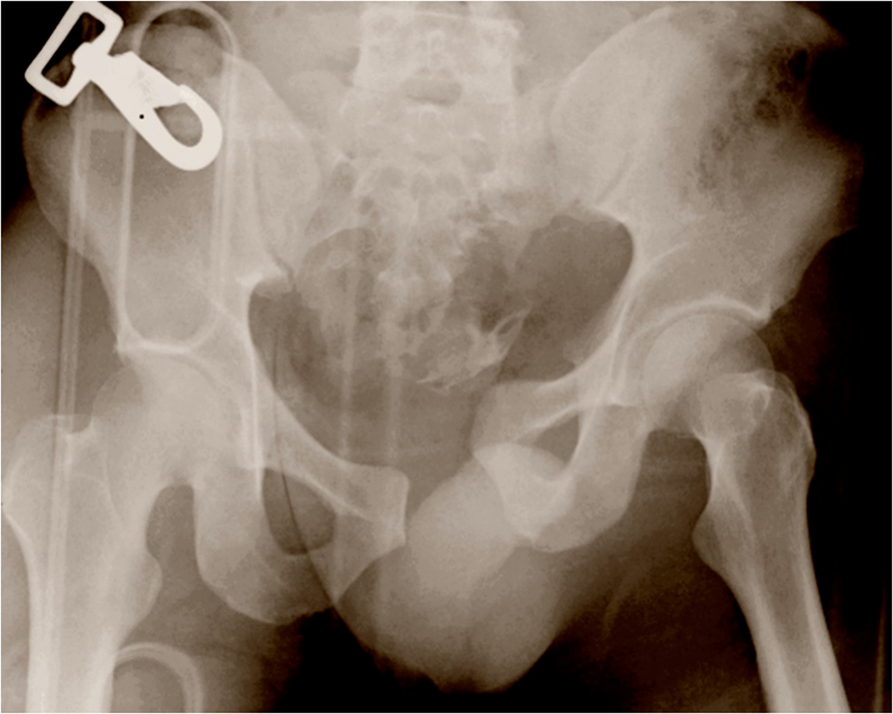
Anteroposterior X-ray of the pelvis showing an unstable pelvic fracture (AO classification type C1-3).
